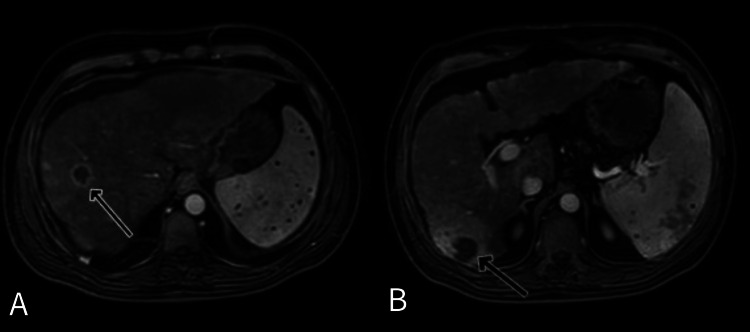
Liver MRI Showing Multiple Liver Masses
